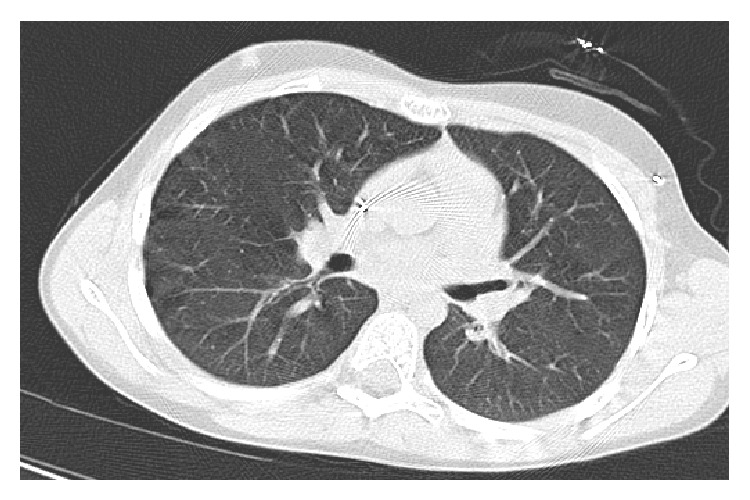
CT scan of the chest prior to start of HSCT. This showed no lung abnormality.
